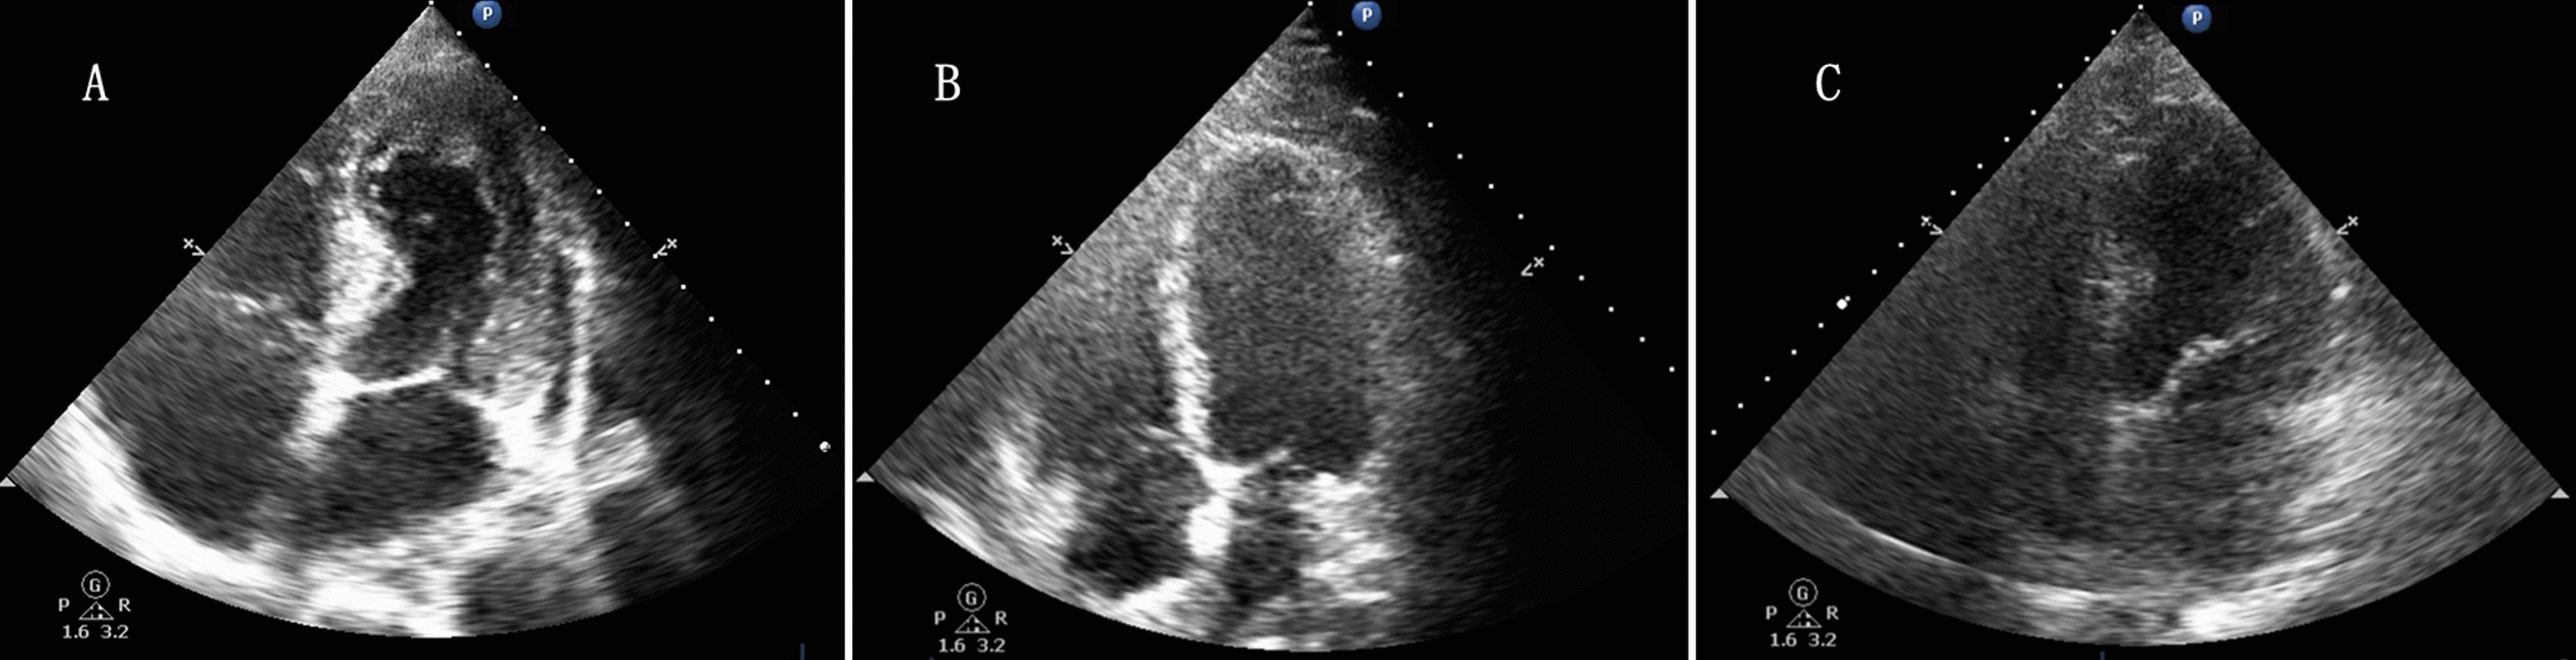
Transthoracic echocardiography of different imaging qualities performed in septic patients. A One patient with a legible image of the entire endocardium (LVEF, MAPSE, GLS and TMAD could be measured accurately); B One patient with an image that was legible for the apex and mitral annulus but indistinct for the LV lateral wall (TMAD and MAPSE could be measured, but LVEF and GLS could not be achieved accurately); C One patient with an indistinct image of the entire endocardium. (None of LVEF, MAPSE, GLS or TMAD could be measured accurately.) LVEF, left ventricle ejection fraction; MAPSE, mitral annular plane systolic excursion; GLS, global longitudinal strain; and TMAD, tissue motion annular displacement
